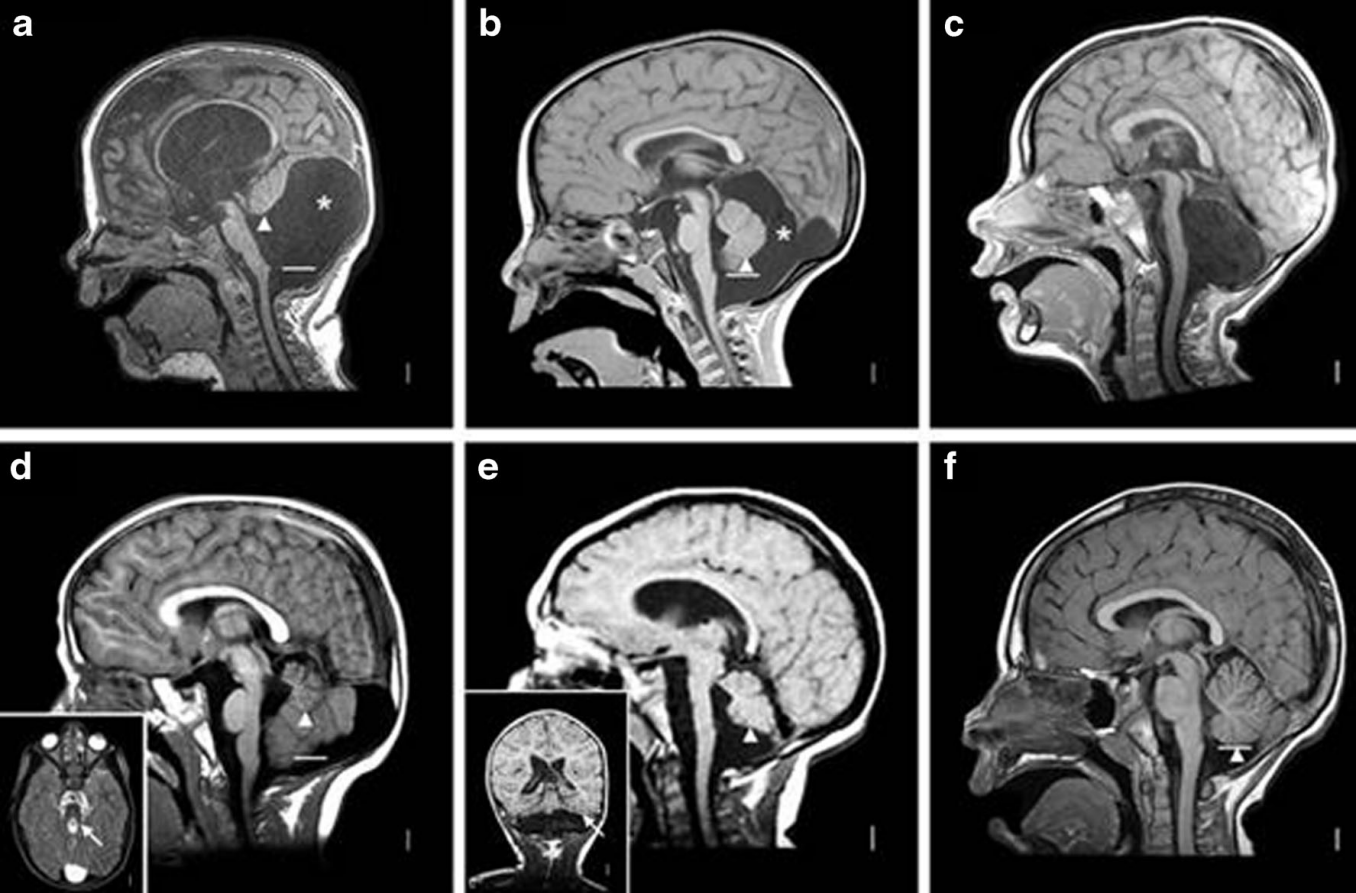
Brain imaging in mid-hindbrain malformations. T1-weighted midline sagittal magnetic resonance images show the key features of classic DWM (a), cerebellar vermis hypoplasia with mega-cisterns magna (b), complete cerebellar agenesis (c), molar tooth malformation seen in JSRD (d), pontocerebellar hypoplasia (e), and normal (f). The *solid white lines* in most images mark the level of the obex, while the *arrowheads* point to the lower edge of the vermis (both landmarks are absent in c). The *asterisk* denotes an enlarged posterior fossa. In (a), the vermis is small and rotated far upwards, the fourth ventricle is enlarged into a cyst-like structure, and the posterior fossa is greatly enlarged causing an elevated tentorium. In (b), the vermis is small but located in the anatomic position, but the posterior fossa is again greatly enlarged. A posterior extension of the cyst appears to scallop the inner table of the skull. In (c), the brainstem is thin without any landmarks other than the tectum, and no cerebellum is seen. In (d), the vermis is very small but located in the correct anatomic position, with portions of the cerebellar hemispheres seen beneath. The *inset* shows the associated “molar tooth” sign (*arrow*). In (e), the brainstem is thin but the obex can just be seen, and the vermis is moderately small. The even more “pancake-like” flattening of the hemispheres is shown in the *inset* (*arrow*)
